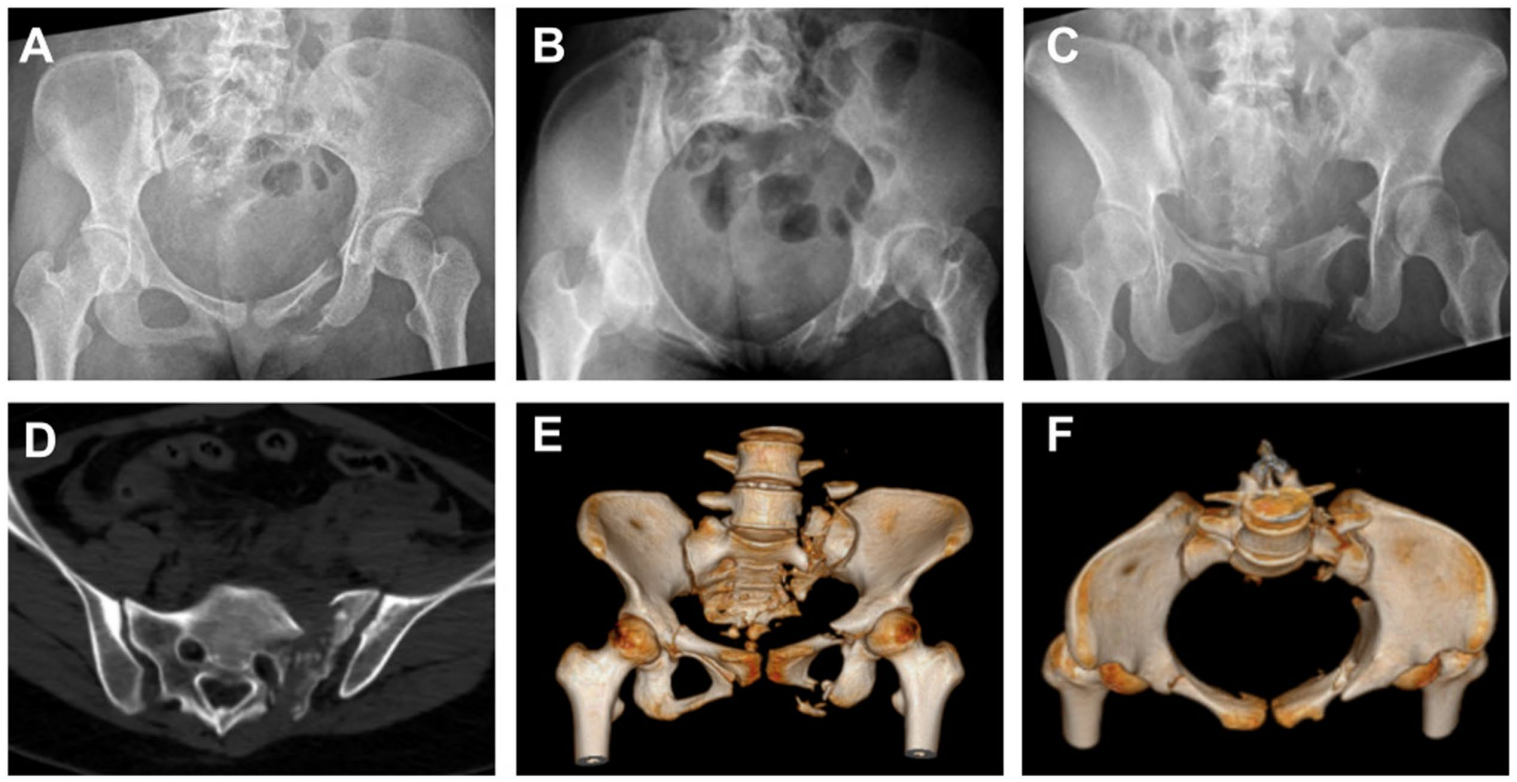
Preoperative imaging studies of the patient’s pelvis were performed using X-rays in the anteroposterior (A), inlet (B), and outlet views (C). The findings suggested a fracture, and CT (D) with three-dimensional reconstruction (E,F) provided more detailed information.
